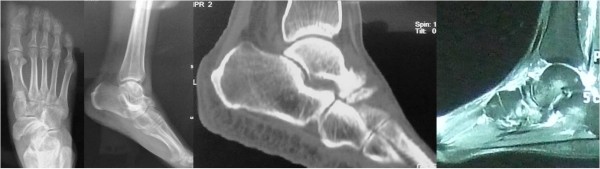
Weight-bearing X-rays, computed tomography (CT) and MRI scans from case 1.
